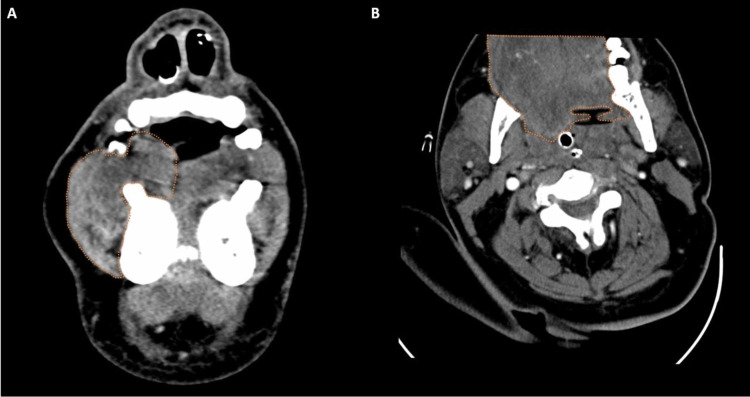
Coronal and axial enhanced CT A: Coronal enhanced CT demonstrating oral tongue and buccal mucosal swelling (orange outline). B: Axial enhanced CT demonstrates oral tongue edema (orange outline) CT: computed tomography
